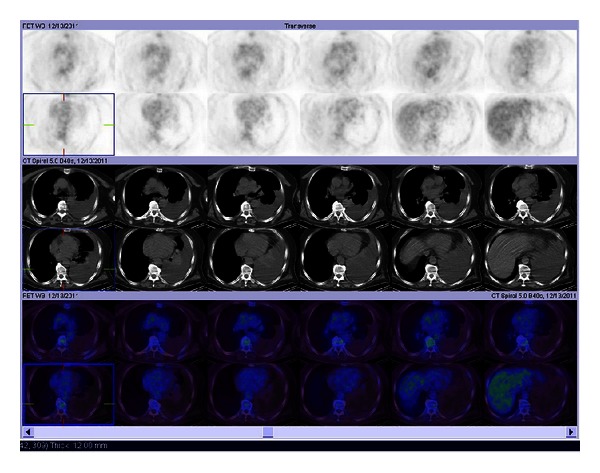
After six cycles of chemotherapy, PET/CT revealed nearly complete response of mediastinal mass.
